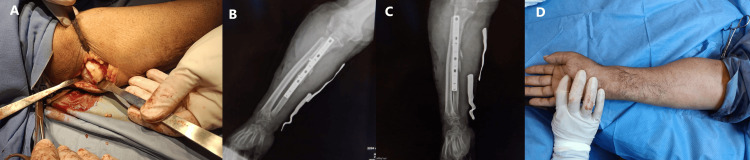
(A) Intraoperative image of radial head exposure showing severe arthritic changes. The radial head and 2 cm of the proximal radius were excised through a direct approach to the dislocated head. (B, C) Anteroposterior and lateral forearm X-rays display ulnar fixation with a fibular graft used to fill the defect, along with radial head excision to facilitate elbow reduction. (D) Postoperative clinical image demonstrating correction of the forearm deformity.
